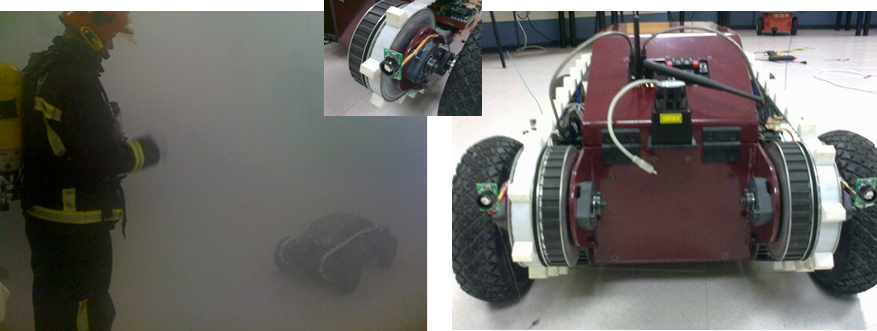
Guardian robot following a firefighter using a combination of radio and ultrasonic sensors.
